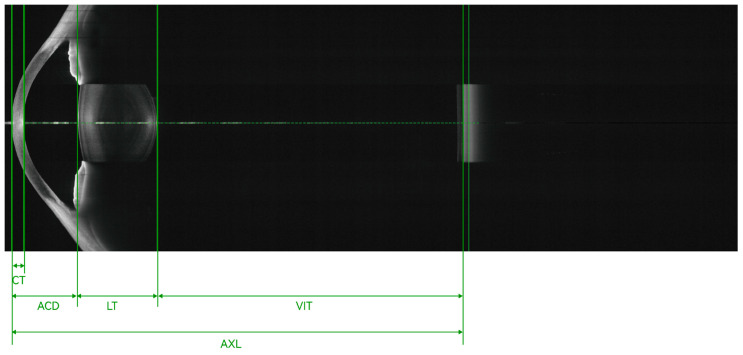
Full-eye, super-depth SS-OCT, achieving an imaging depth up to 40 mm and a 16 mm field of view. CT, cornea thickness; ACD, anterior chamber depth; LT, lens thickness; AXL, axial length; VIT, vitreous.
